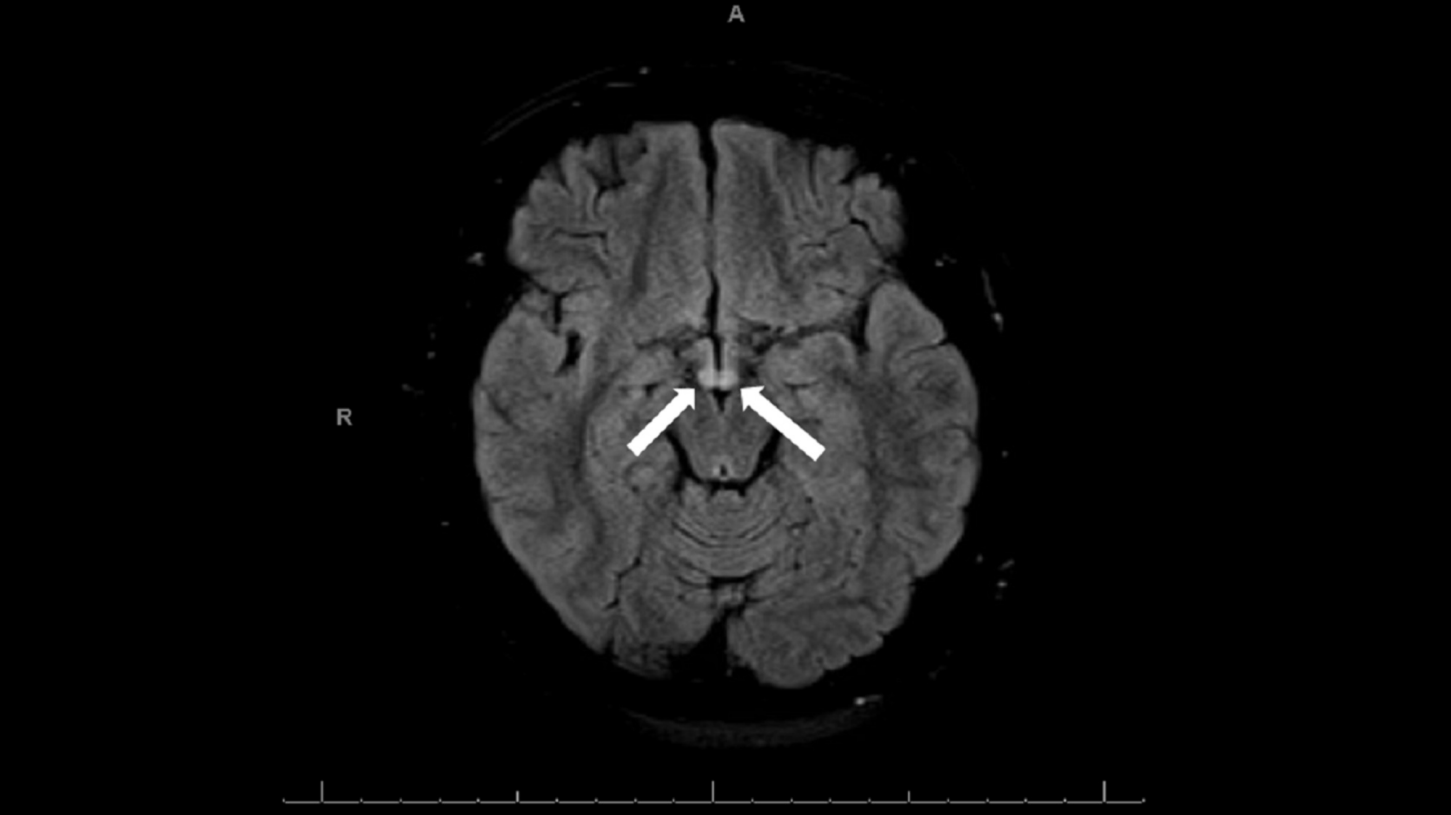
MRI of the brain with and without contrast showed increased signal on T2 FLAIR imaging in the mammillary bodies bilaterally, and enlargement of both mammillary bodies. The radiologist's interpretation was that these findings can be seen in Wernicke’s encephalopathy. FLAIR: Fluid-attenuated inversion recovery; MRI: Magnetic resonance imaging.
